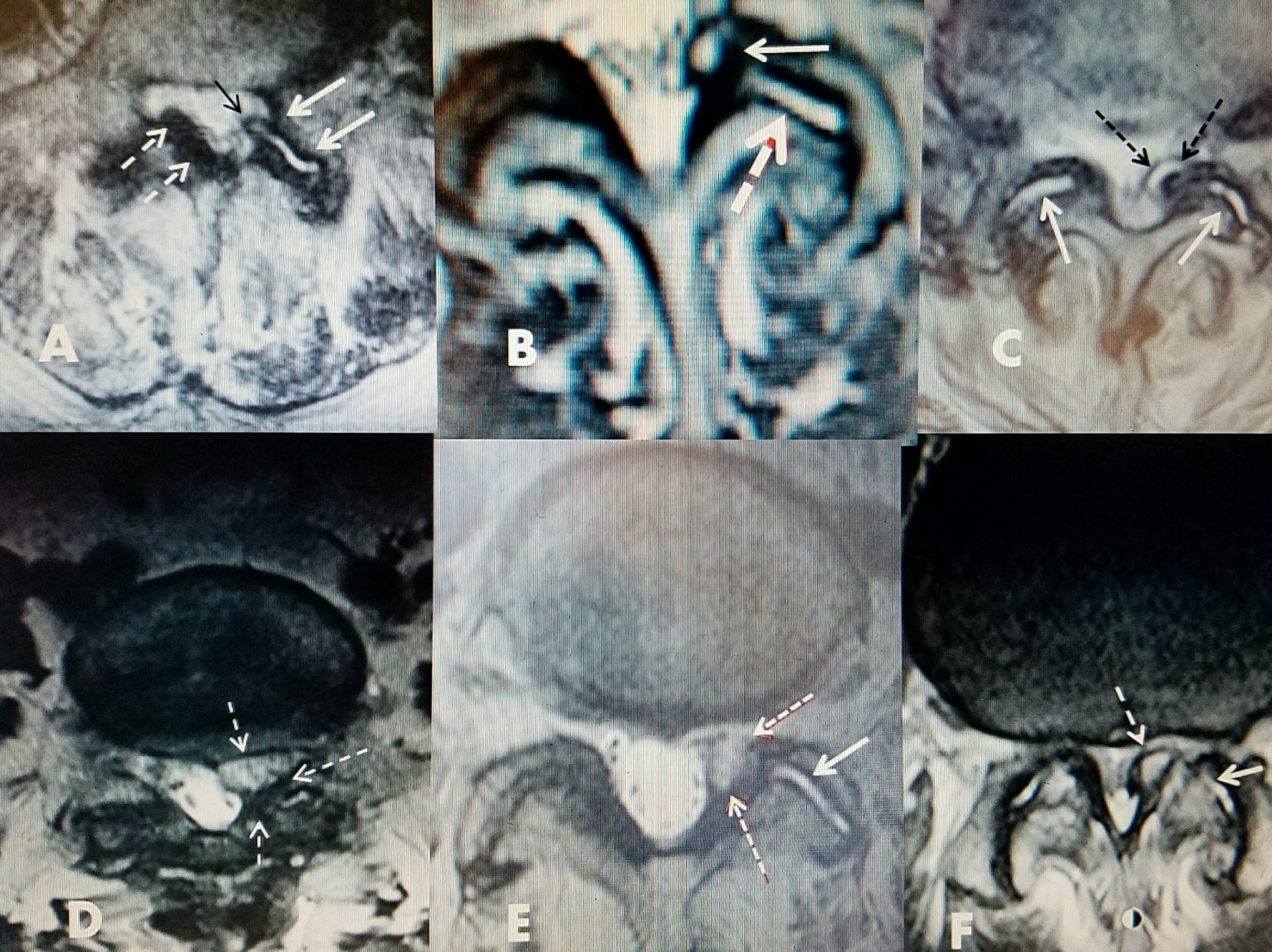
Axial MRI scans showing the different fluid densities within the facet cysts Examples of different MRI characteristics of different lumbar facet cysts. A: Mixture of posterior ligament stenosis (dashed white arrows), development of fluid in facet joint (white arrows), and beginning of cyst on same side extending out of the ventral joint space into the lateral recess (black arrow). B: Unilateral facet and foraminal cyst at L4-5 causing lateral recess stenosis (upper white arrow). High intensity T2 signal change indicates fluid within joint (dashed white arrow). C: Bilateral hyperintense fluid in both facet joints (solid white arrows) shows cyst formation on one side with medial encroachment into lateral and dorsal spinal canal (dashed black arrows). D: Foraminal cyst with more homogenous fluid on MRI that is more gelatinous (dashed white arrows). E: Hyperintense signal of fluid in facet joint (white arrow) and more gelatinous isodense fluid in facet cyst (dashed red/white arrows). F: Fluid in facet joint (white arrow) and bone spur from inferior facet mimicking cyst (dashed arrow).
